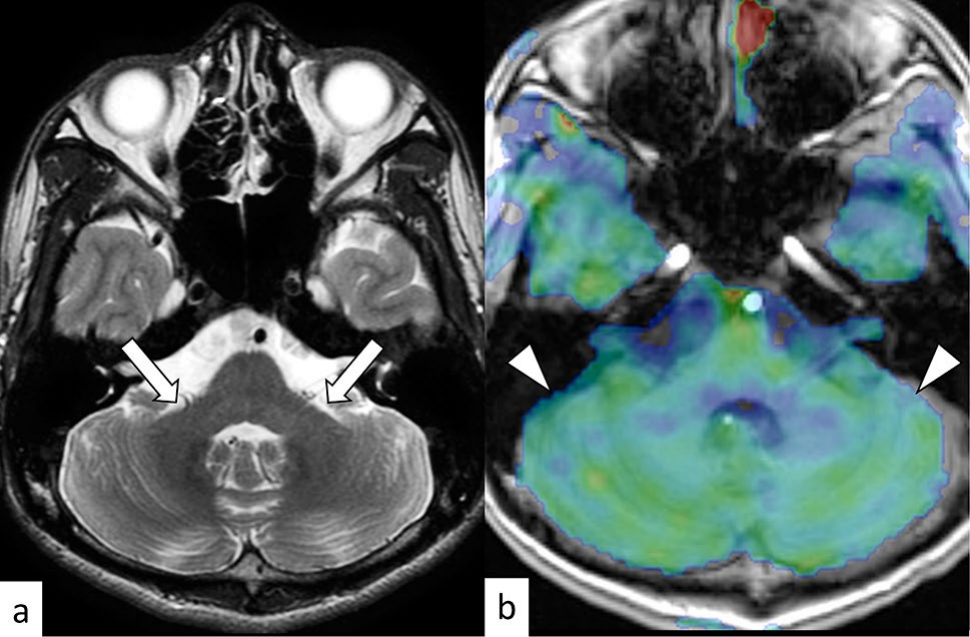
A 21-year-old woman with SCA7. T2-weighted axial image shows mild cerebellar atrophy (a, arrows). Arterial spin labeling image shows decreased blood flow in the cerebellum (b, arrowheads)
